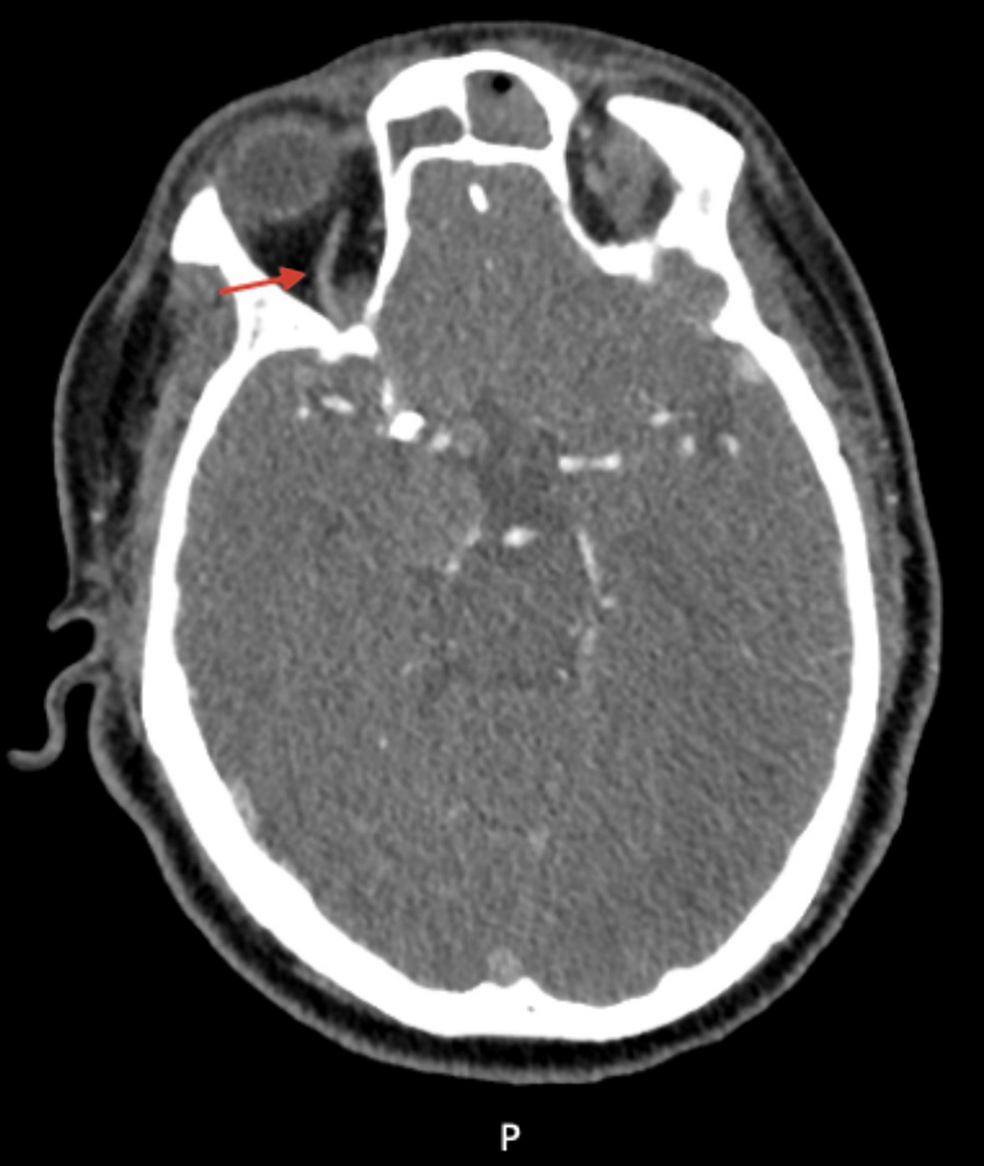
CT angiogram of the head after flow diverter placement showing a patent right ophthalmic artery (red arrow)
